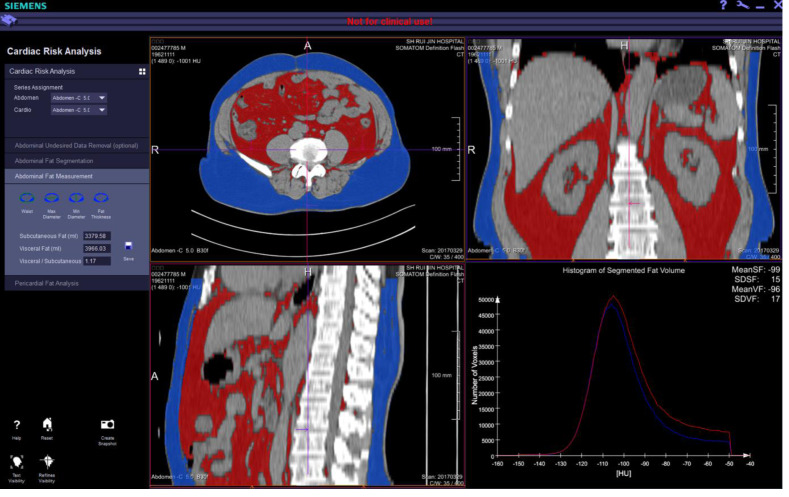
The intra-abdominal fat volume (IFV) measured by multi-detector rows computed tomography (MDCT) obtained on comprehensive view at different levels in coronal, sagittal and cross-sectional images with multiplanar reconstruction (MPR) images.
